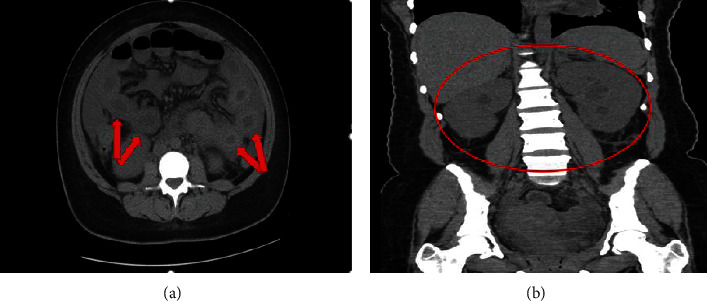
Abdominal CT scan showing small bowel thickening (a), bilateral hydroureteronephrosis (b), and moderate intra-abdominal ascites.
